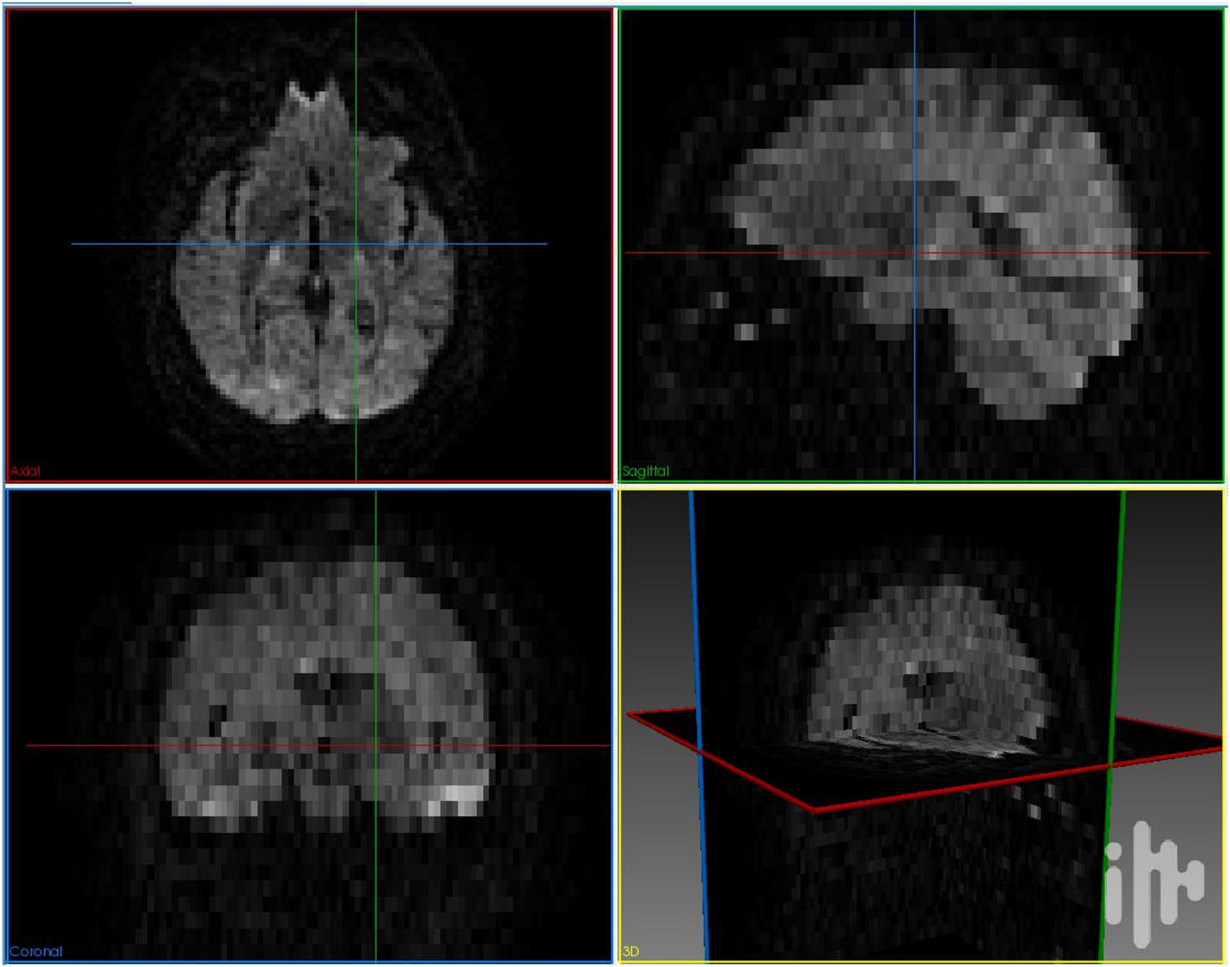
Example of a raw DWI sample that did not pass manual QC because of an acquisition protocol error.
